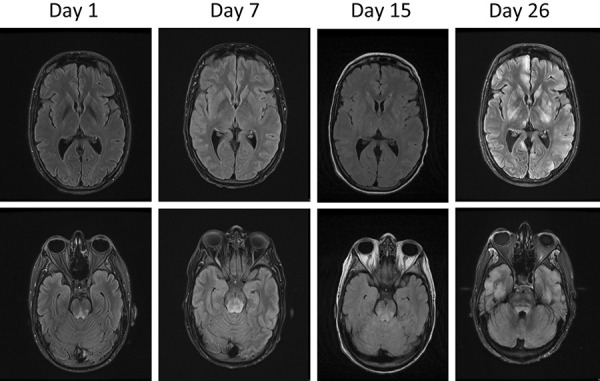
Brain MRI axial FLAIR (fluid-attenuated inversion recovery) sequence findings at day 1, 7, 15, and 26 after ICU admission showing severe progression of signal alterations in the brainstem, cortical areas, and basal ganglia.
